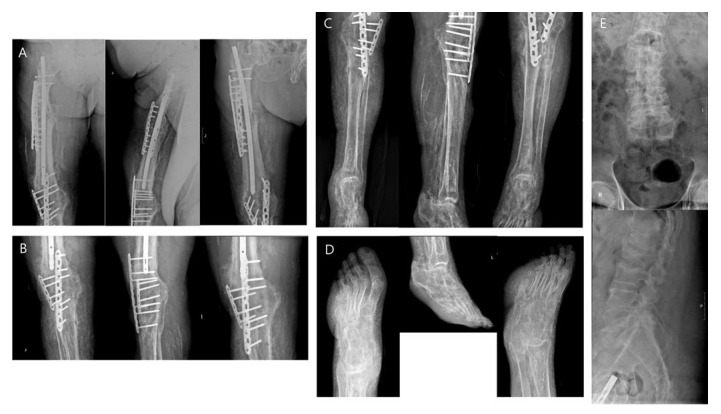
A 32-year-old male was referred to the Department of Nuclear Medicine to evaluate long-standing left leg pain. About 7 years ago, he had left femur shaft, patella, and tibia fractures in a car accident and had a history of undergoing open reduction and internal fixation. Moreover, about 5 years ago, a nonunion of the left proximal tibia fracture caused knee floating, and it was managed with knee fusion using a Charnley clamp. A year ago, he suffered multiple injuries in a car accident, including a left distal femur fracture. The fractures were managed with open reduction and internal fixation with a bone graft. He complained of persistent pain in his left lower leg despite multiple surgeries. Over the years, he has taken many plain radiographs, including of the left lower extremity. These studies demonstrated diffuse osteopenia, pseudocyst lesions, a moth-eaten appearance to the trabecular pattern, several radiolucencies, a permeative pattern, an old fracture, and multiple soft tissue calcifications (Figure 1). Plain radiographs of the left femur (A), left knee (B), left tibia (C), left foot (D), and lumbar spines (E). Plain radiographs of the lower limb were of the anterior view, lateral view, and oblique view in order from left to right, and in the case of the L-spine, the anterior view and lateral view were arranged in order from the top. In all plain radiographs of the left lower extremity, numerous abnormal calcified opacities of various sizes were observed in soft tissue. Additionally, it showed diffuse osteopenia, several radiolucencies, or a permeative pattern. The left first toe showed a pseudocystic lytic lesion on its medial side. Deformities were also in the left fourth and fifth toes. The L-spine radiograph revealed spondylolytic spondylolisthesis of L5 on S1, degenerative disk disease of the lumbar spine, and compression fracture at the L1 and L2 spines.
